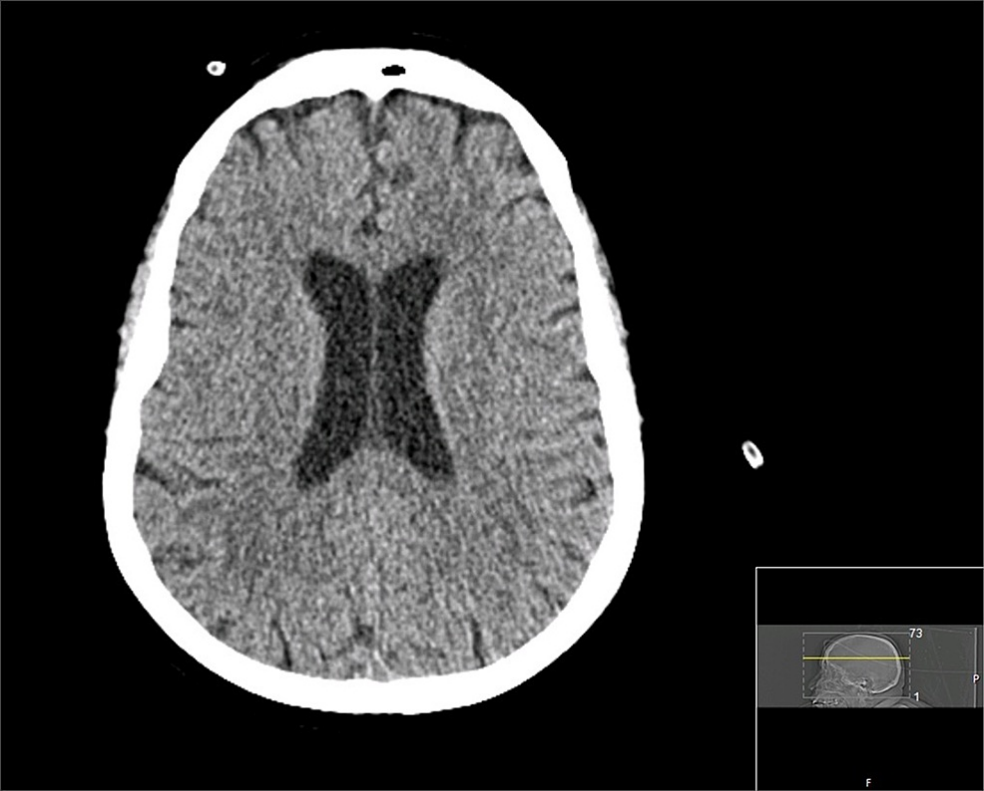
Axial brain CT scan revealing no cerebral insult
